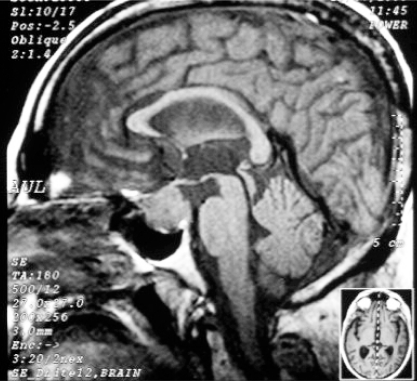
Sagittal section of MRI brain showing pituitary tumour
